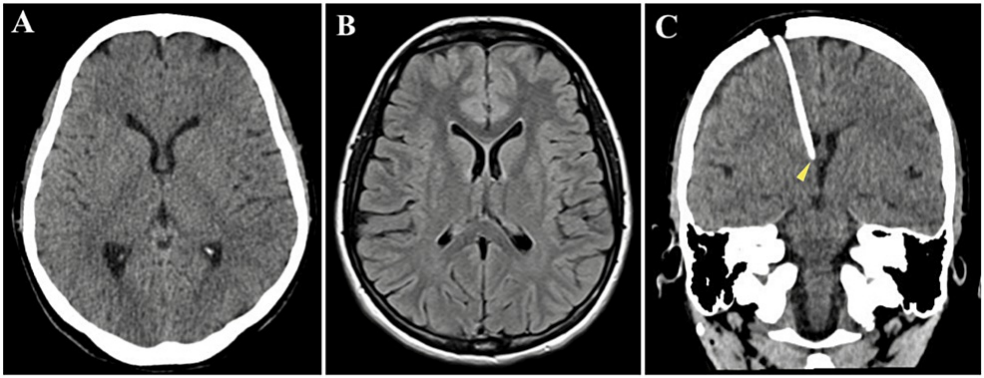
Case 1 Axial brain CT scan (A) and MRI (B) show a slit-like ventricle pattern. (C) Postoperative coronal CT scan shows accurate ventricular catheter position in a sufficient pool of CSF with the tip in the foramen of Monro (yellow arrowhead).
